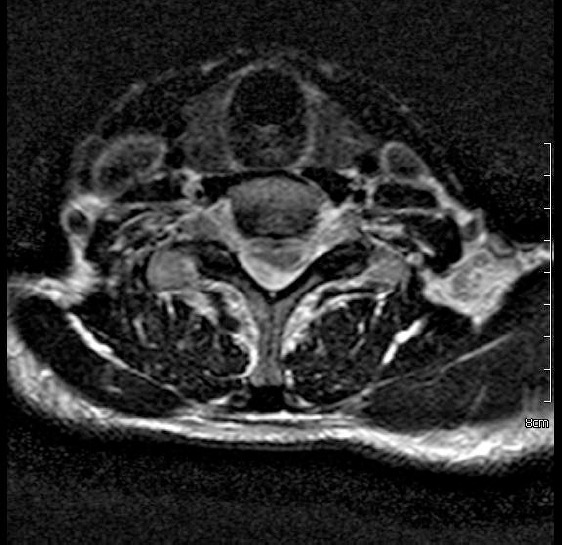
A cystic mass is located right posterolaterally to the spinal cord in preoperative T2 MRI axial section*.
